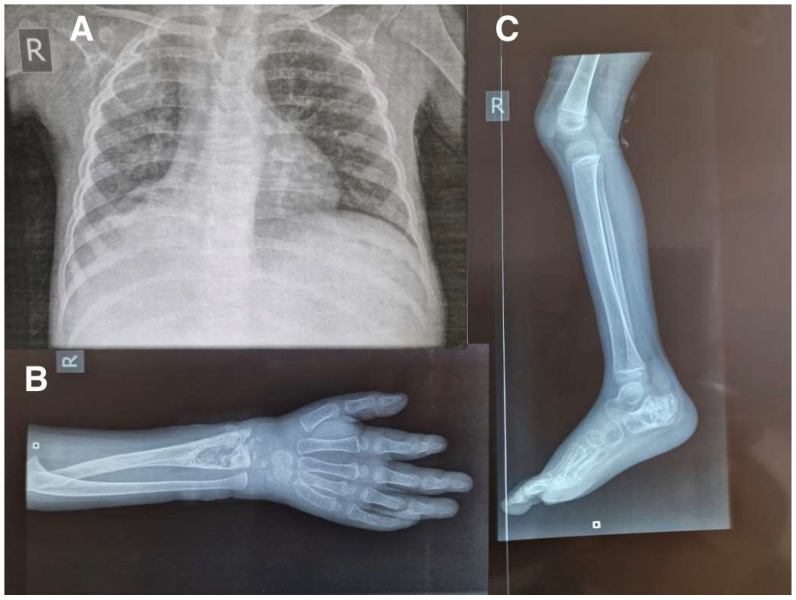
The chest x-ray (A), X-ray of the right forearm (B) and the X-ray of the right leg (C).
